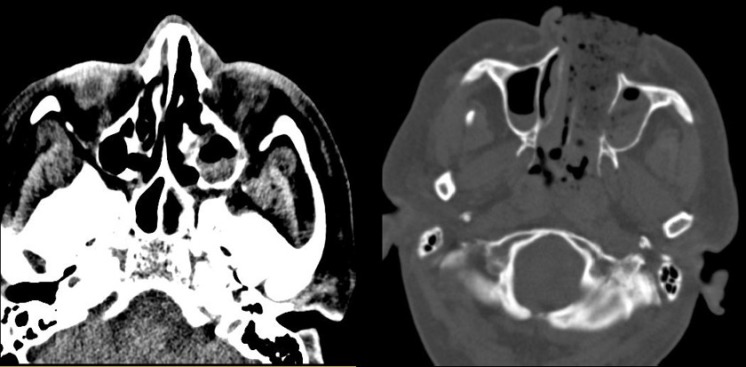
Brain computed tomography scan revealing the involvement of the left maxillary, ethmoidal sinuses, and nasopharynx and the destruction of the bone of the medial wall of the left maxillary and ethmoidal sinuses
